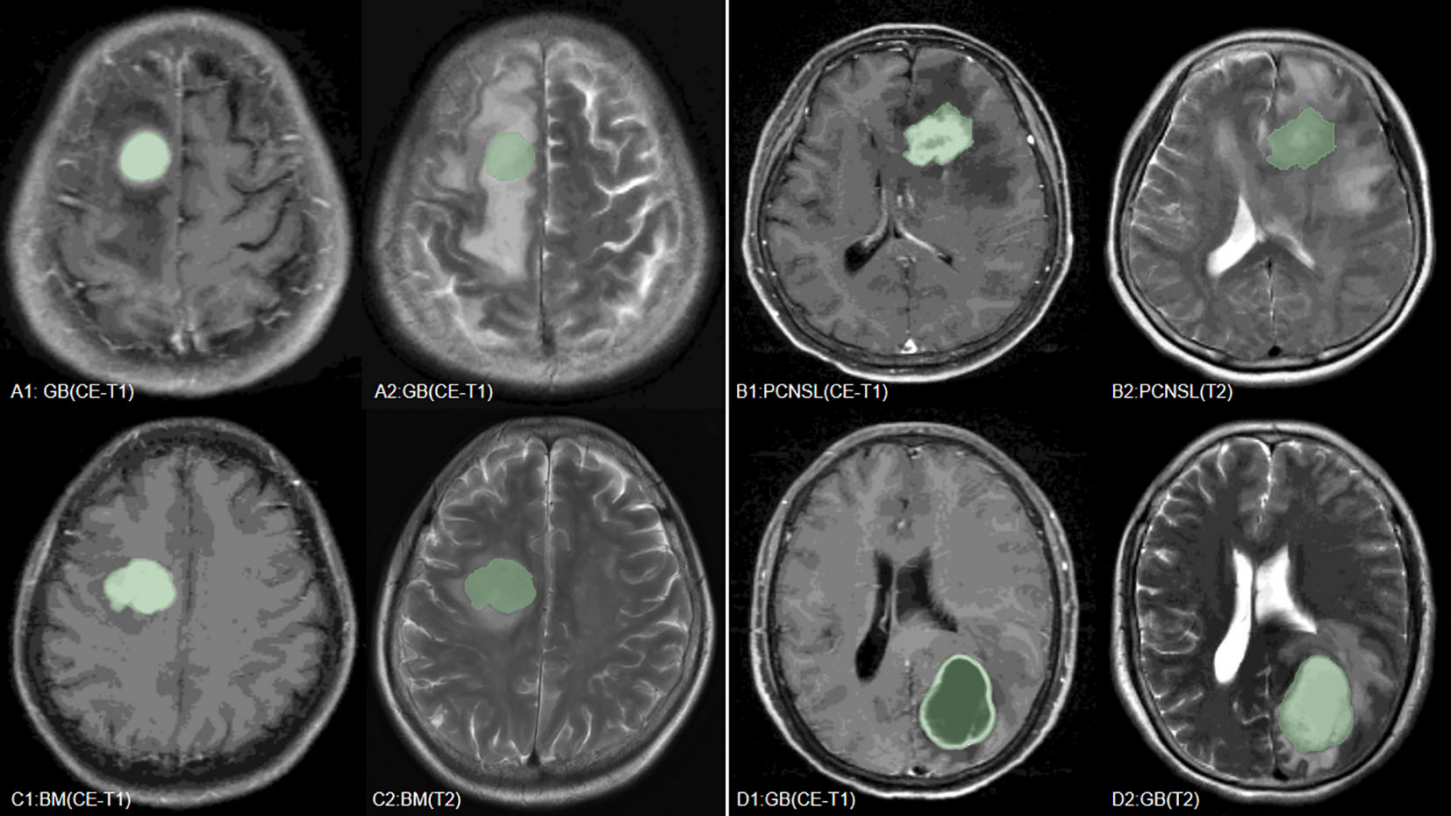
We have listed MRI images of four cases, where (A–C) are the GB, PCNSL, and BM correctly predicted by the model, respectively, and (D) is a case where the model incorrectly predicted BM as GB.
